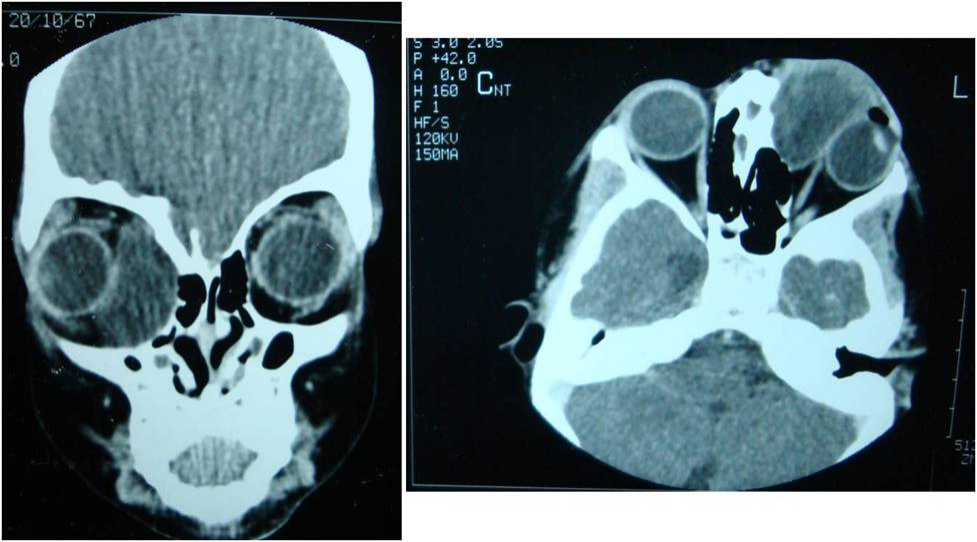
Computed tomography of head and orbit showing a hypodense extraconal mass in the left orbit with no intracranial connectivity.
